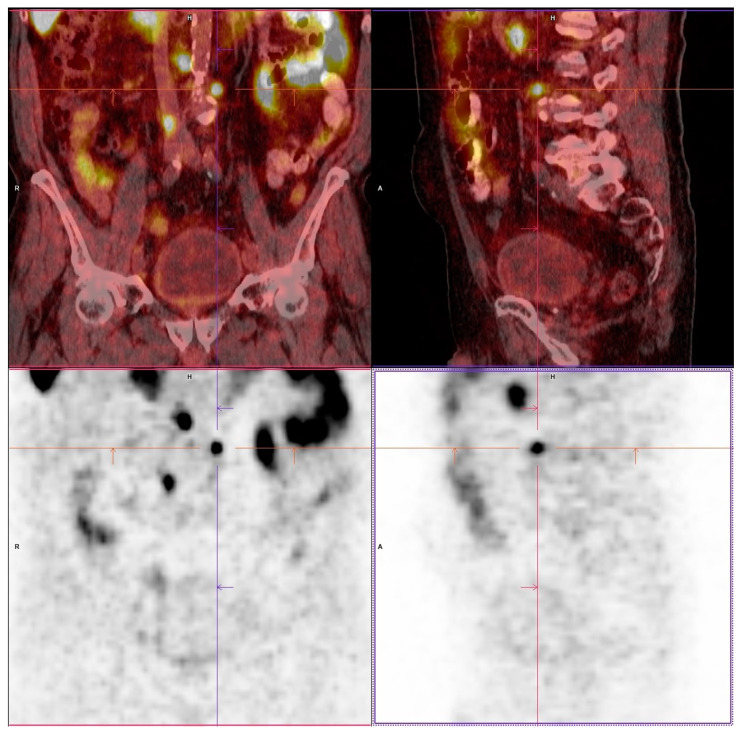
F-18-PSMA-1007 imaging demonstrating a paraaortic metastatic suspicious finding 1 year after local therapy of the primary tumor and increasing PSA-level. Top with combined coronal and sagital CT and PET images, bottom with coronal and sagital PET images.
